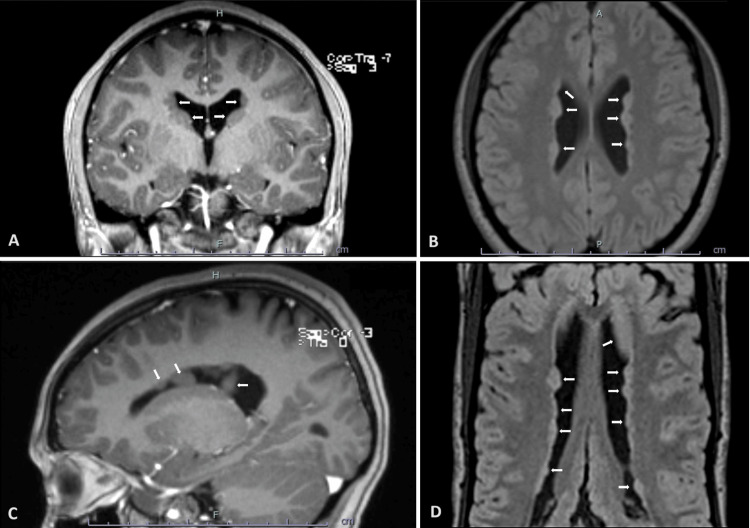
Patient’s head magnetic resonance imaging. Images of our patient’s head magnetic resonance imaging, on coronal (A), axial (B and D) and sagittal (C) planes, showing bilateral subependymal nodular irregularities lining the lateral ventricles. These nodules exhibit a similar signal evolution to that of the grey matter, confirming the diagnosis of periventricular nodular heterotopias.
